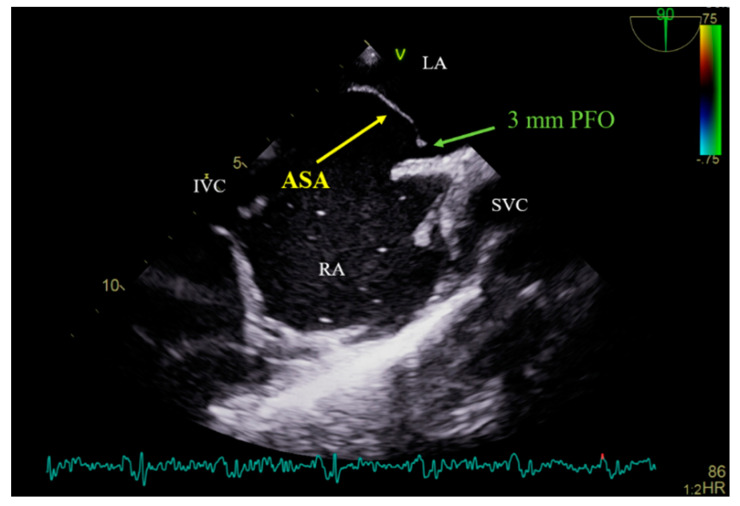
Bicaval view TEE showing PFO with an atrial septal aneurysm. TEE—transesophageal echocardiography; PFO—patent foramen ovale; ASA—atrial septal aneurysm; LA—left atrium; RA—right atrium; IVC—inferior vena cava; SVC—superior vena cava.
